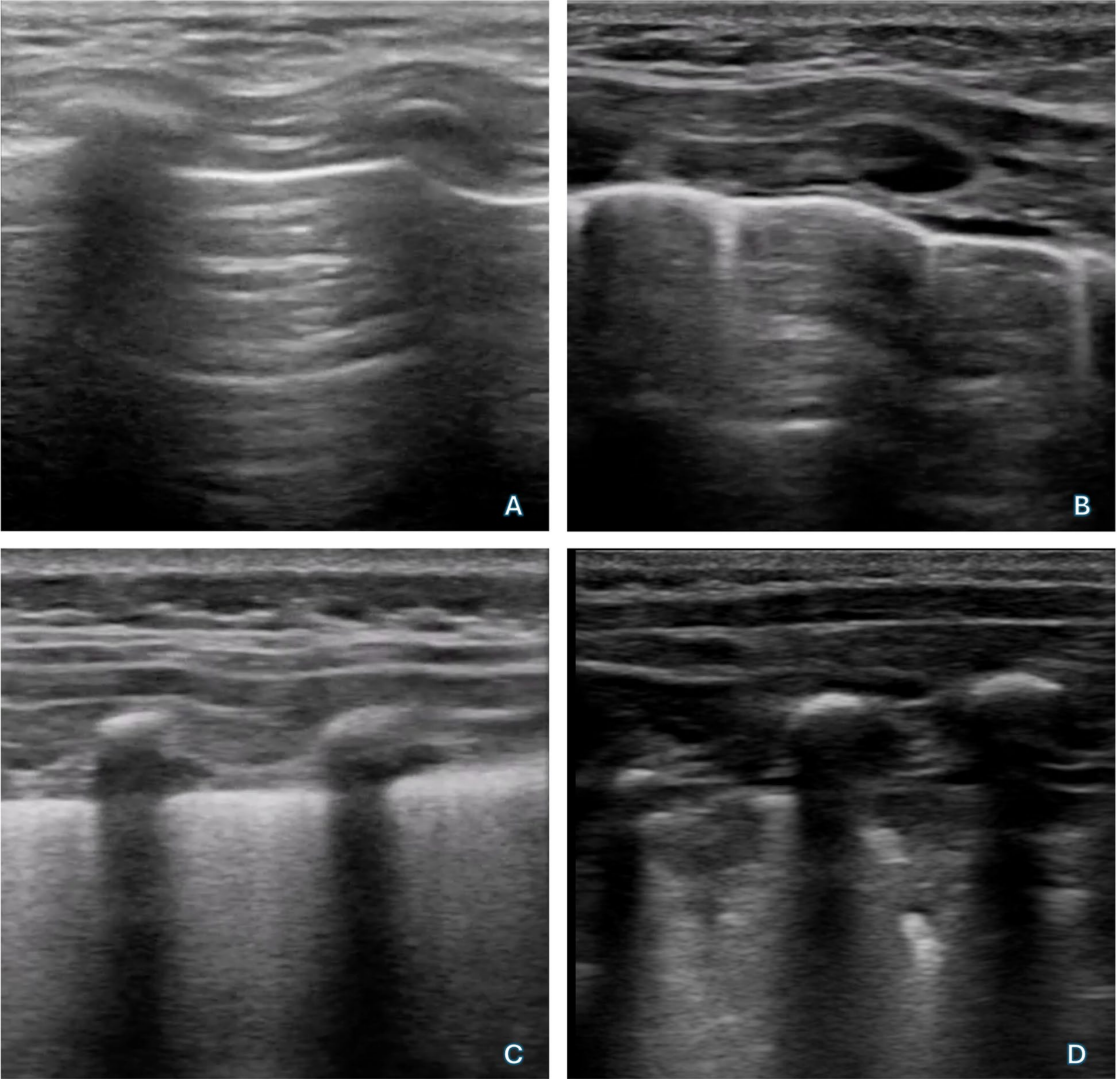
Ultrasound classification. A Normal aeration, A-lines or less than 2 B-lines with lung sliding [score 0]; B: mild alveolo-interstitial pattern—3 or more well-spaced B-lines with lung sliding [score 1]; C severe alveolo-interstitial pattern, represented by multiple, crowded, and coalescent B-lines occupying more than 50% of scanned intercostal space (i.e., “white” lung) with lung sliding [score 2]; D severe loss of aeration—tissue-like pattern or consolidation [score 3]
